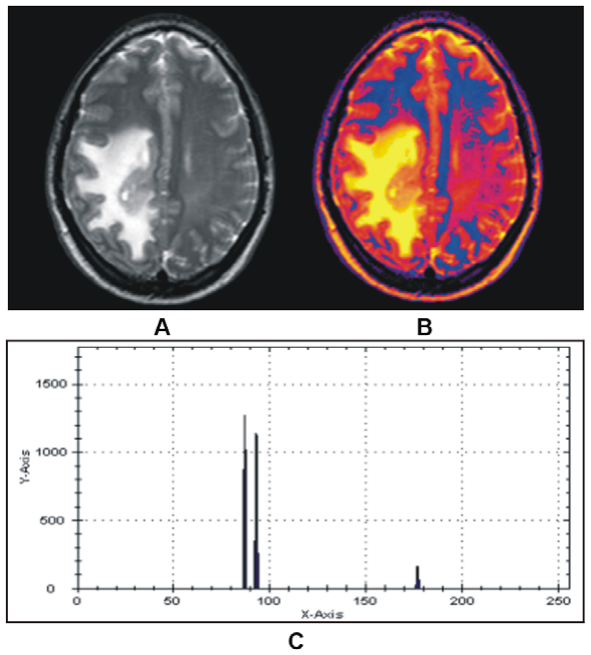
Color representation of T2 brain MR image with our proposed colorization method. (A) Abnormal T2 brain MR image of patient aged 32. (B) Color transformed image. (C) Selected centroids with proposed method.
